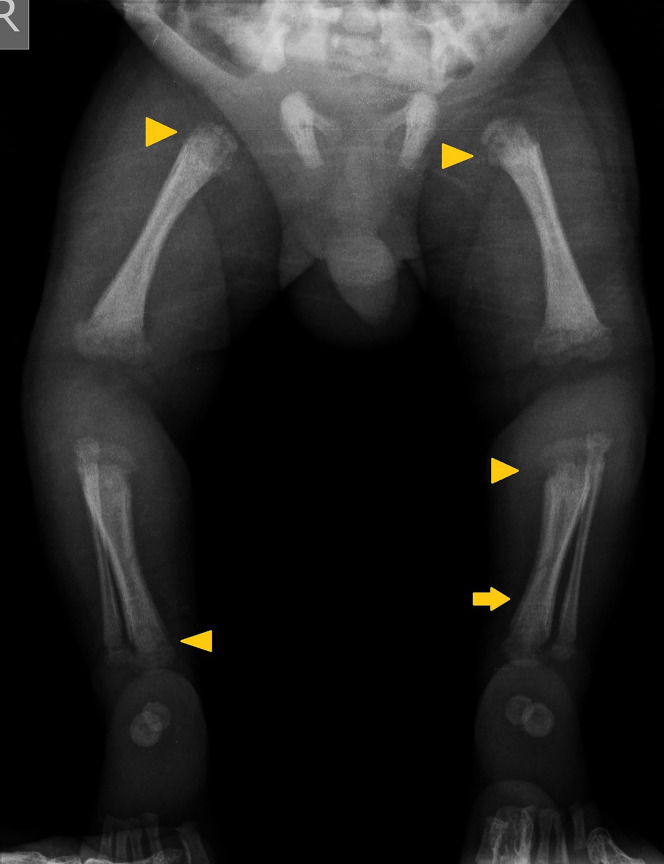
Radiographic evidence of periostitis and metaphyseal bands in lower extremity long bones.
